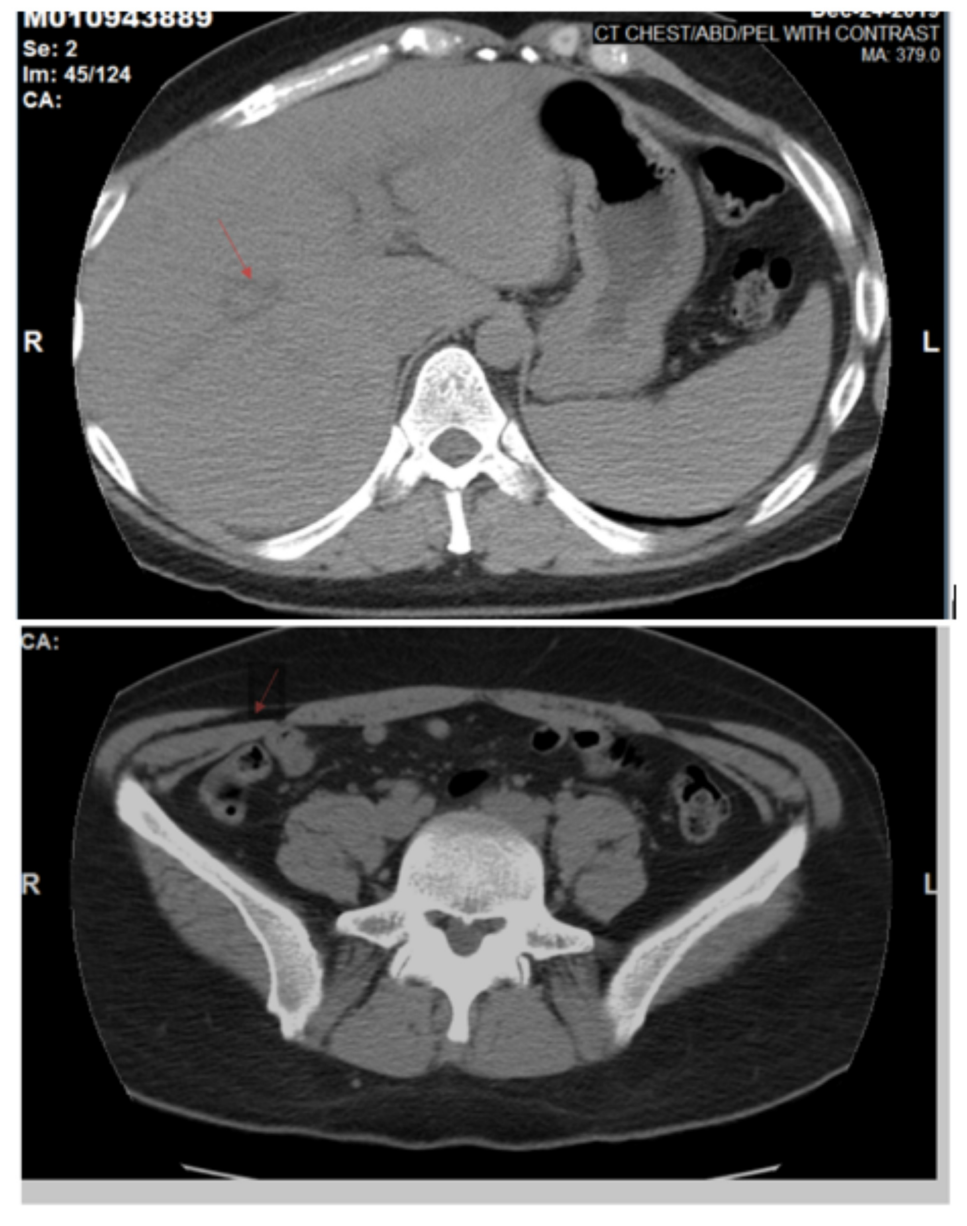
CT abdomen (top) and pelvis (bottom) revealed periportal edema with free fluid in the pelvis suggestive of an infectious and/or inflammatory process such as gastroenteritis
